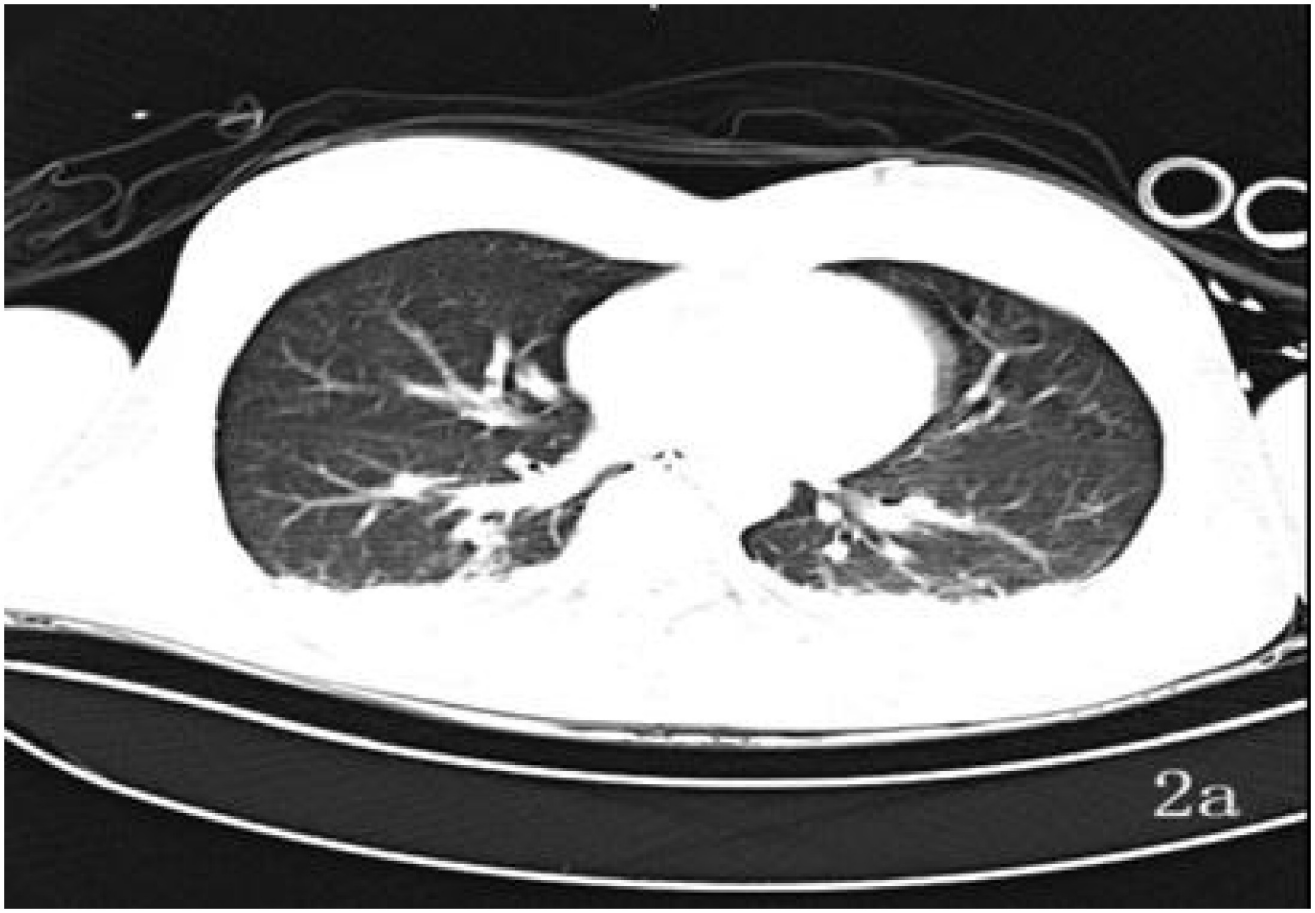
Thoracic CT (a): 13 June–bilateral pulmonary infiltrates and pleural effusions present.
